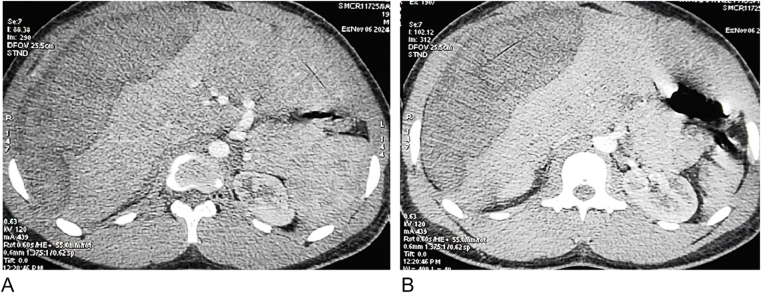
(A and B) Follow-up contrast-enhanced computed tomography (CECT) of the abdomen (early arterial phase), performed 2 months after presentation (6 November 2024), shows further interval resolution of the hepatic subcapsular hematoma with no new hemorrhage.
